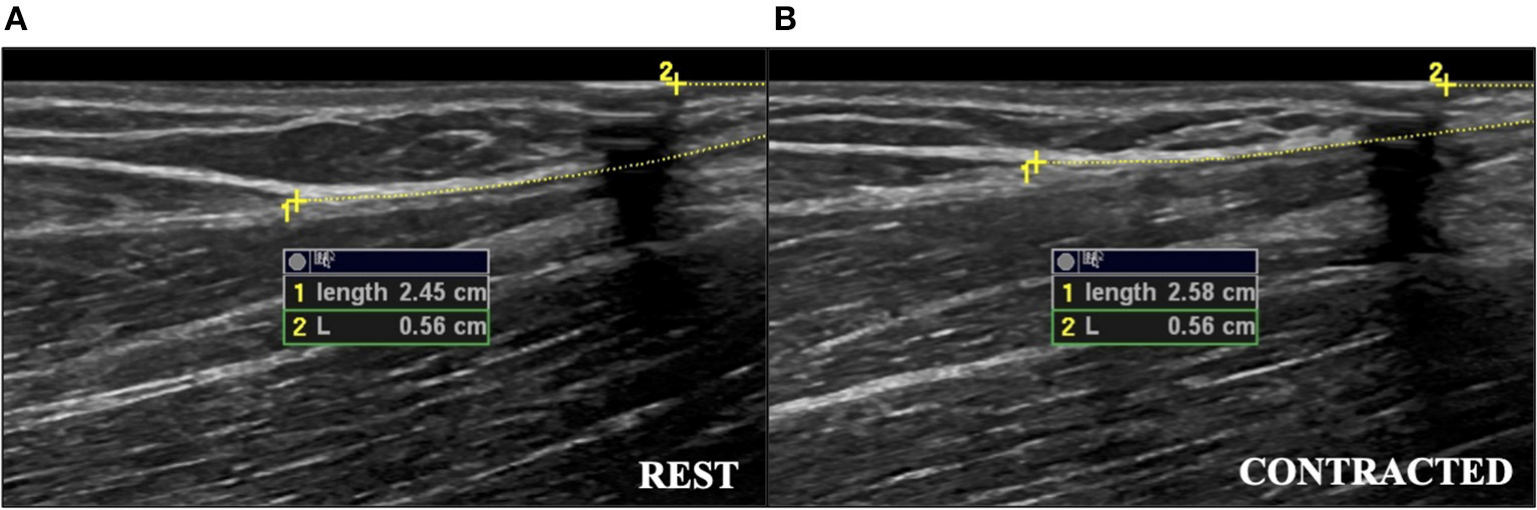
The ultrasound measurements of tendon elongation in a young female during a 25% MVC contraction. (A) Measurement at rest of distance from muscle-tendon junction to edge of ultrasound field of view (Length 1), and measurement of distance from hyperechoic marker to the edge of ultrasound field of view (L2). (B) Measurement during the contracted state. The difference between the measurements during the contracted and resting states was used as the measure of tendon elongation. Images have been cropped for reproduction, but analysis captured the full length of the tendon. MVC, maximal voluntary contraction.
